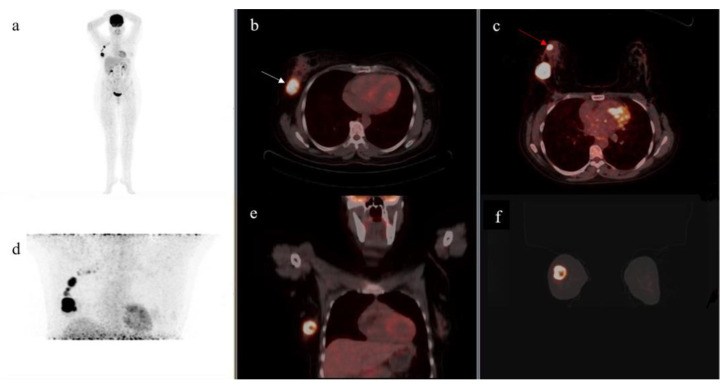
MIP (a,d) showing FDG avid lesions in the right breast with axillary lymph node metastasis. Fused axial PET/CT images in supine (b) showing a lesion (white arrow) in the right breast, which is better delineated on prone (c) with the visualization of an additional lesion (red arrow). Fused coronal PET/CT cuts of supine (e) and prone (f) acquisition are displayed.
